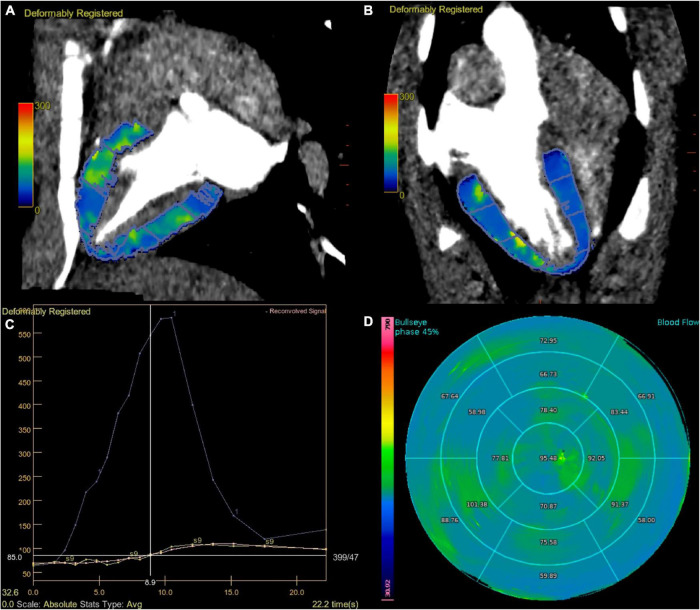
Dynamic perfusion acquisition at 70 kV. Fused MBF map and CT images, divided according to AHA segments (A,B). The graph view displays the AIF (displayed in blue), TAC of the myocardium (displayed in yellow), and reconvolution curve (displayed in pink). A smooth fitting curve (pink line) was obtained through the TAC of each segmental myocardium (yellow line) (C). Bull’s-eye map with quantitative MBF values, based on 70 kV of stress state (D). AHA, American Heart Association; AIF, artery input function; TAC, time attenuation curve.
